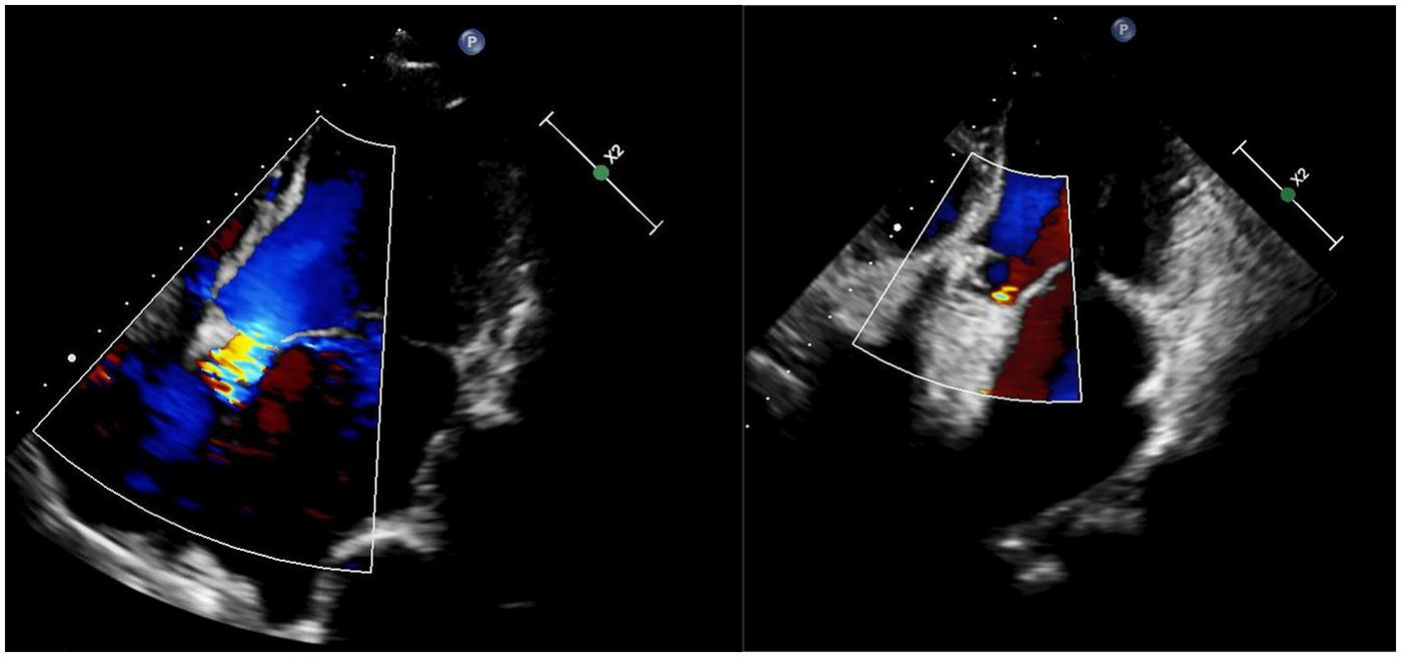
Echocardiograms before (left) and after (right) the procedure.
